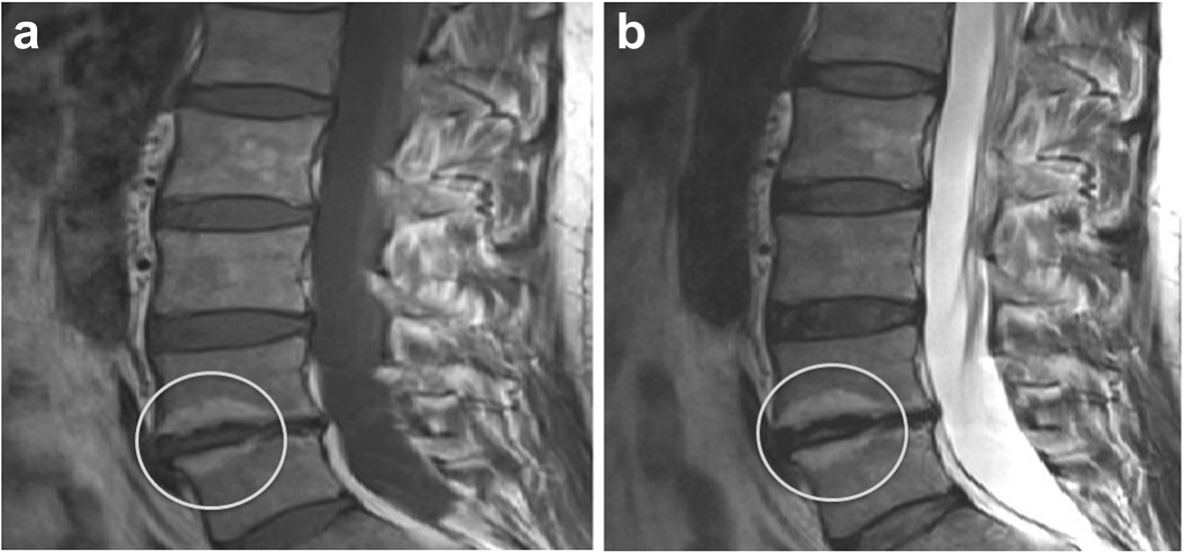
An example of Modic type 2 change in the vertebrae adjacent to the L4/5 intervertebral disc, which also demonstrates reduced disc height. a – T1-weighted image, b – T2-weighted image
